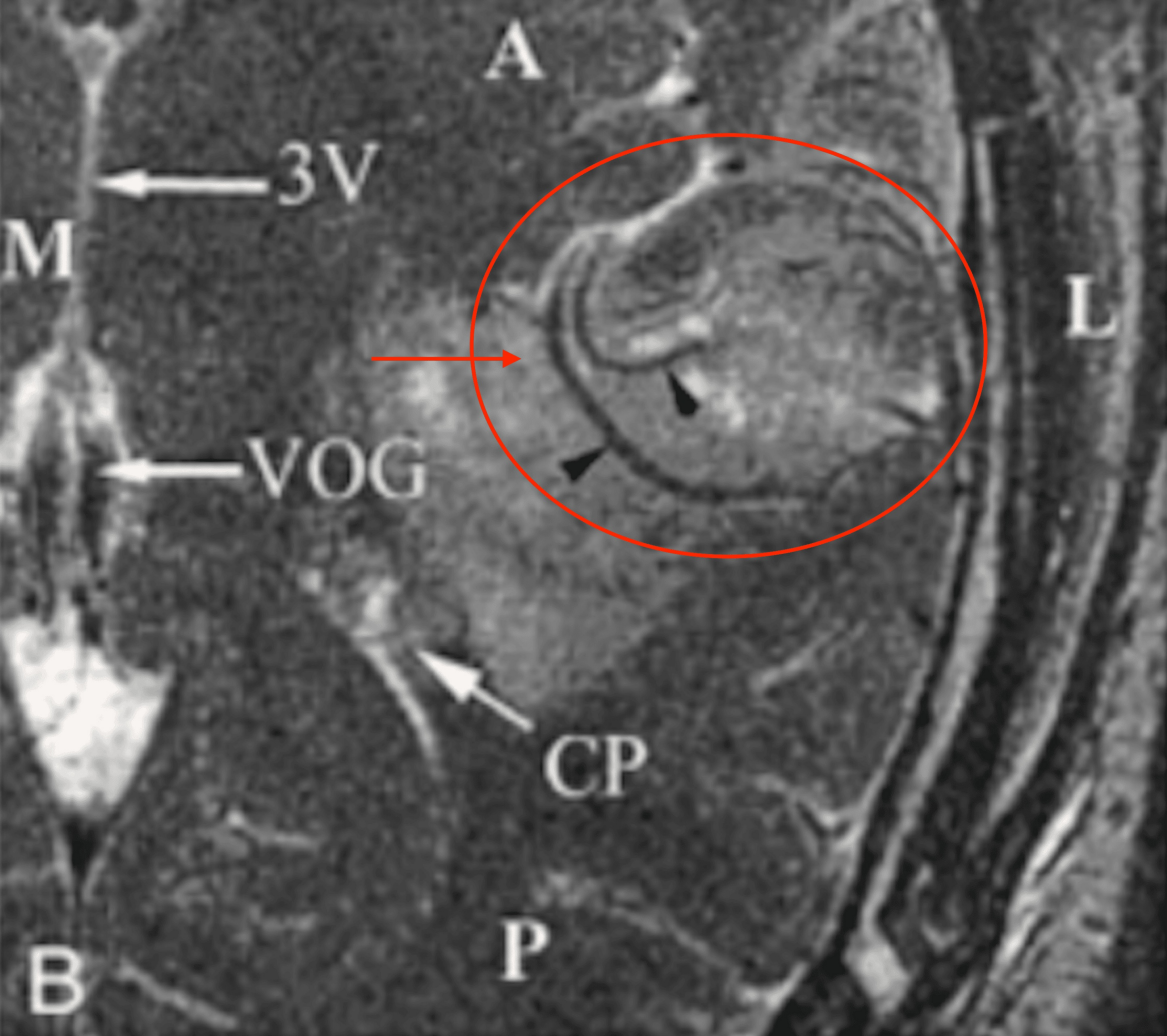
Increased angiogenesis seen in patients with glioblastoma using T2 MRI CP: choroid plexus; VOG: vein of Galen; 3V: third ventricle. Image Source: Christoforidis et al., 2002 [8]; Used with permission from the American Society of Neuroradiology.
